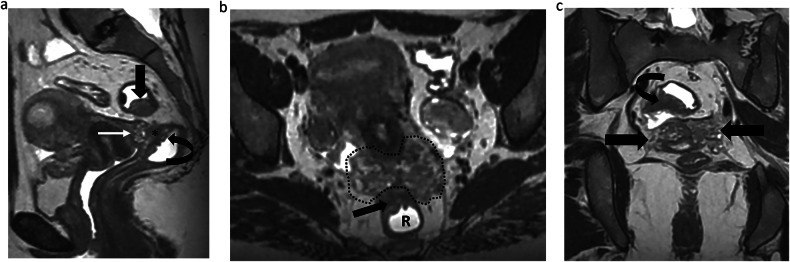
Endometriosis in a 36-year-old woman with deep dyspareunia and chronic constipation. a Sagittal T2-weighted image shows a retrocervical nodule (white straight arrow) involving posterior vaginal wall associated with obliteration of the pouch-of-Douglas (*) (mediocentral compartment) and rectal infiltration (curved arrow) (posterocentral compartment). Another nodule affecting the rectosigmoid colon is noted (black straight arrow). b, c Axial (b) and coronal (c) T2-weighted MR images show bilateral mediolateral parametrial extension (area inside the dashed line in (b) and straight arrows in (c), inferring involvement of the inferior hypogastric plexus. Bowel involvement is also noted (straight arrow in b and curved arrow in c). Thus, this patient is quoted with a dPEI score of 5 (severe disease) with 4 compartments affected (mediocentral, posterocentral, right mediolateral, left mediolateral)
